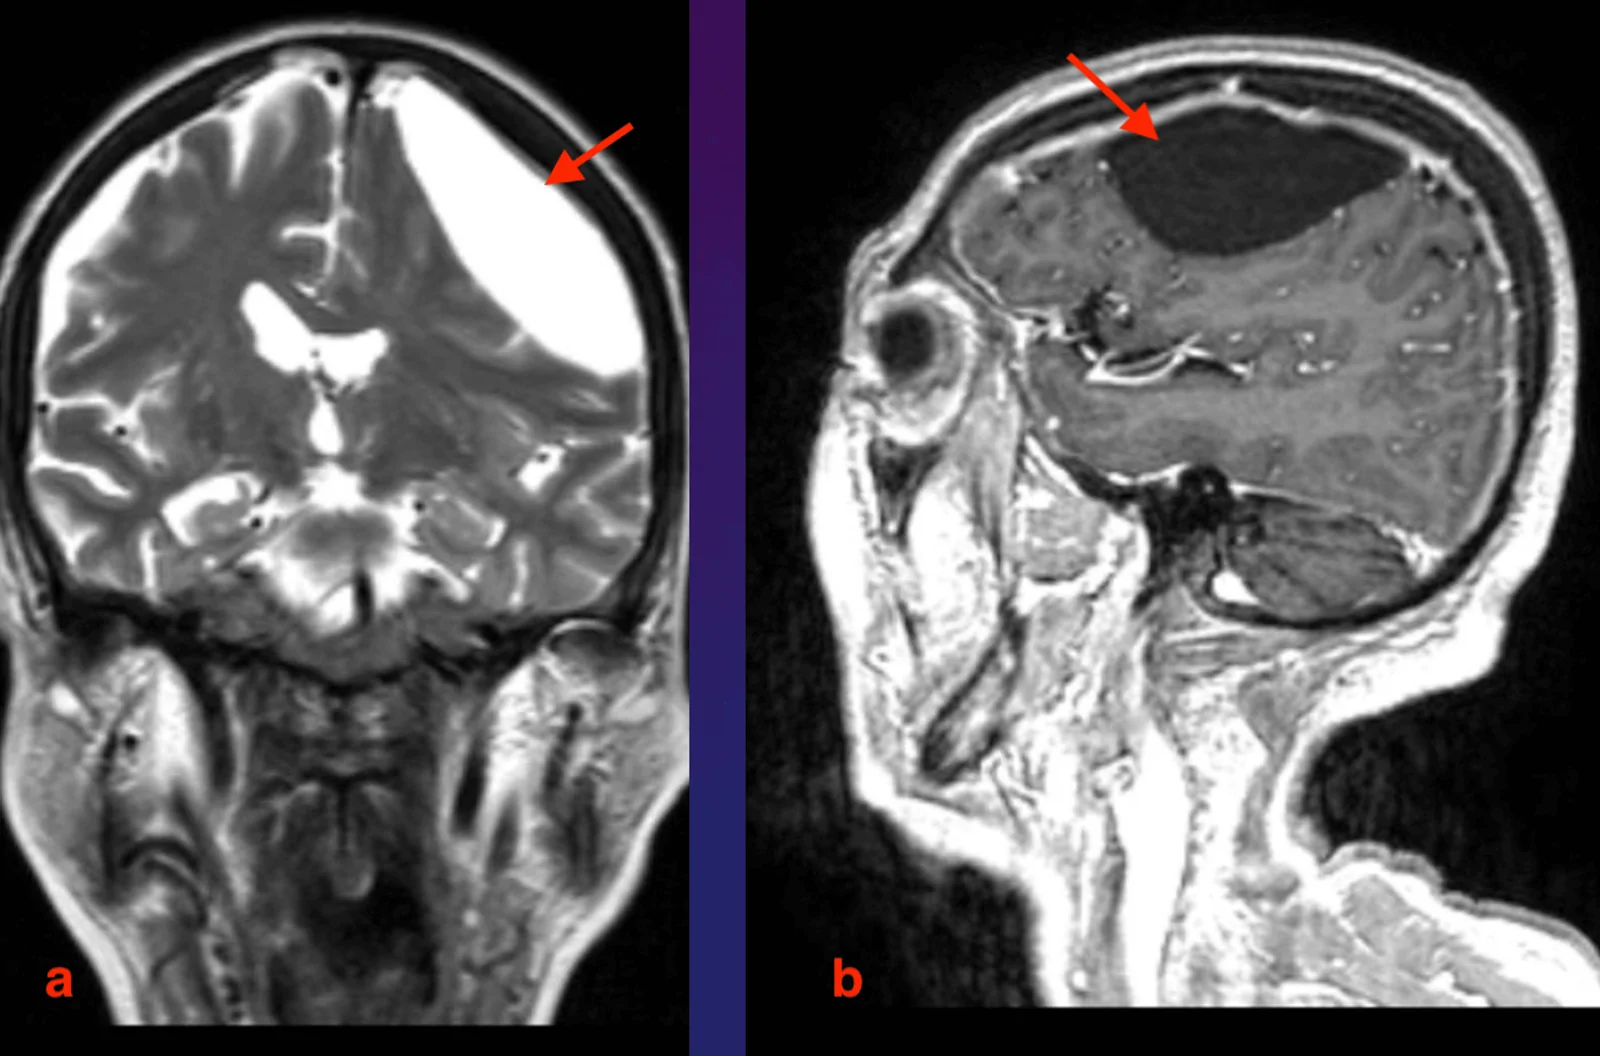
Cranial MRI: (a) Coronal T2-weighted view showing a left frontal hyperintense cystic lesion (red arrow) with displacement of the brain and the ipsilateral lateral ventricle. (b) Sagittal view with contrast medium showing dural enhancement in addition to the hypointense cystic lesion (red arrow).
